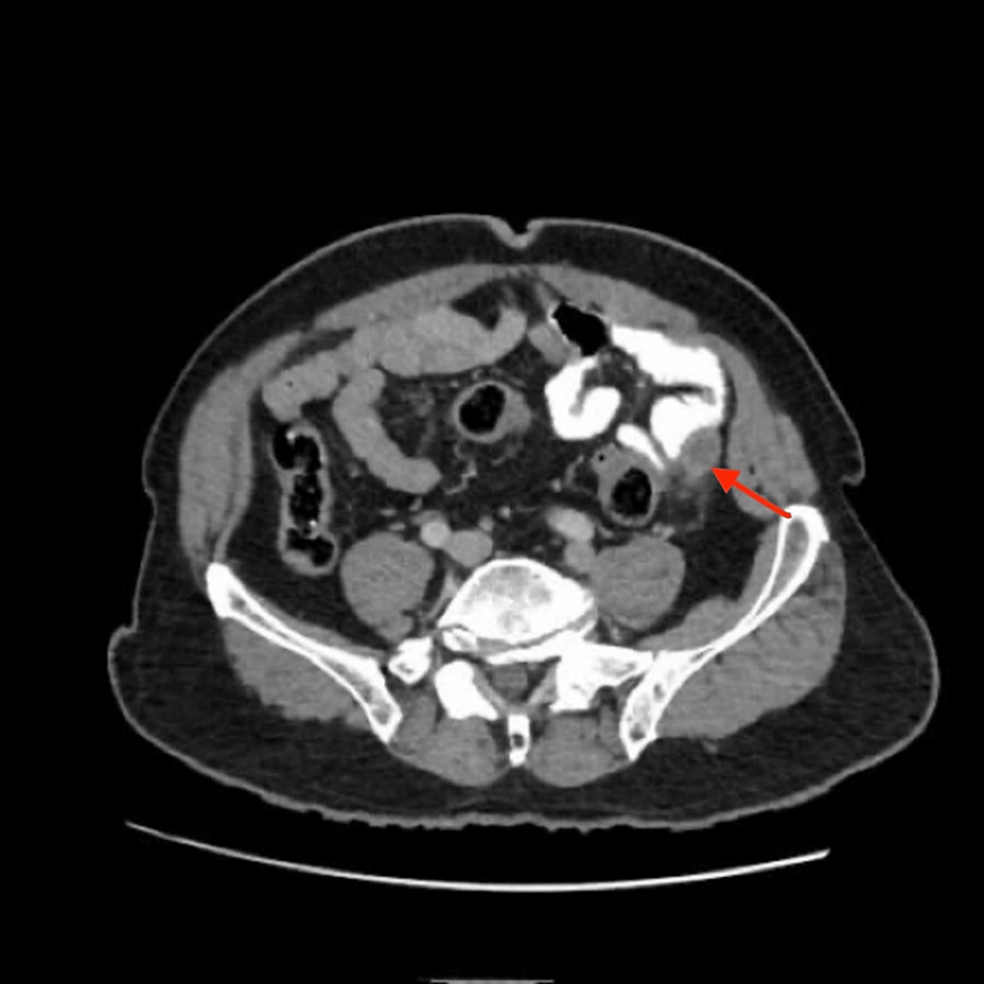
CT scan of the abdomen showing a swollen appendix in the left iliac fossa with adjacent fat stranding (red arrow)
